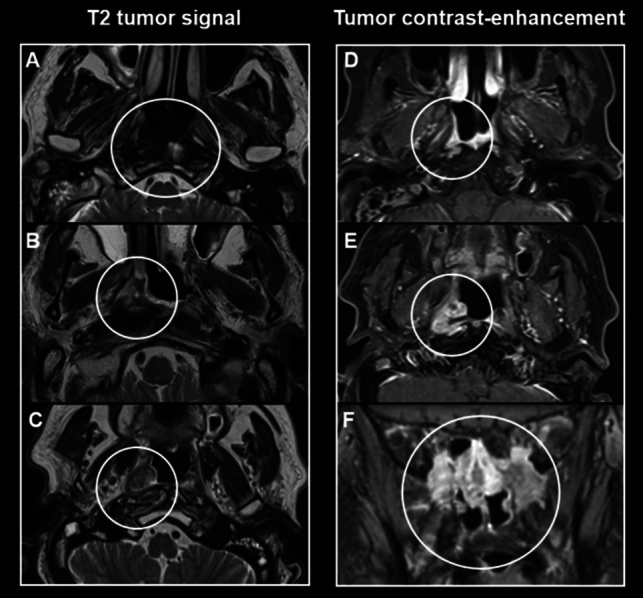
Examples of nasopharyngeal carcinoma features (white circles) after radiation therapy in T2-weighted images (A, C, E) and contrast-enhanced T1-weighted images (B, D, F). B, D and C, E from the same patients, respectively. A Hypointense prevertebral soft tissues, representing a scar. B Hyperintense soft tissue in the right pharyngeal recess, representing edema. C Intermediate hyperintense (“evil grey”) soft tissue in the right pharyngeal recess, representing tumor recurrence. D Thin linear mucosal enhancement in the right pharyngeal recess, representing inflammation. E Soft tissue with focal contrast enhancement, representing tumor recurrence. F Soft tissue with extensive contrast enhancement in the skull base foramina and perineural regions, representing tumor recurrence
